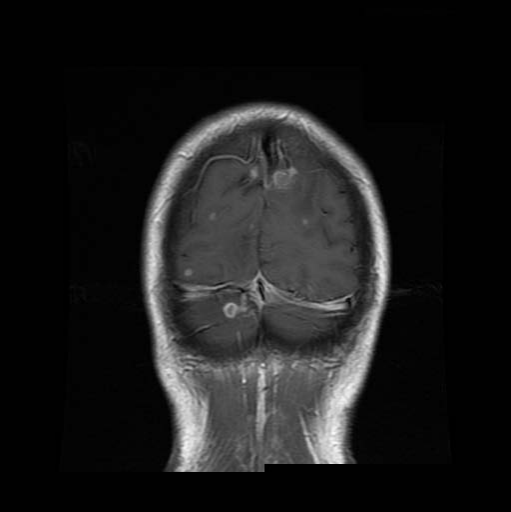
T1 weighted magnetic resonance imaging of the head showing multiple foci of contrast enhancement (abscesses). Scan taken during the second admission showing lesions suspicious of abscesses near the grey-white junction of both cerebral hemispheres, and a small enhancing lesion in the right cerebellar hemisphere.
